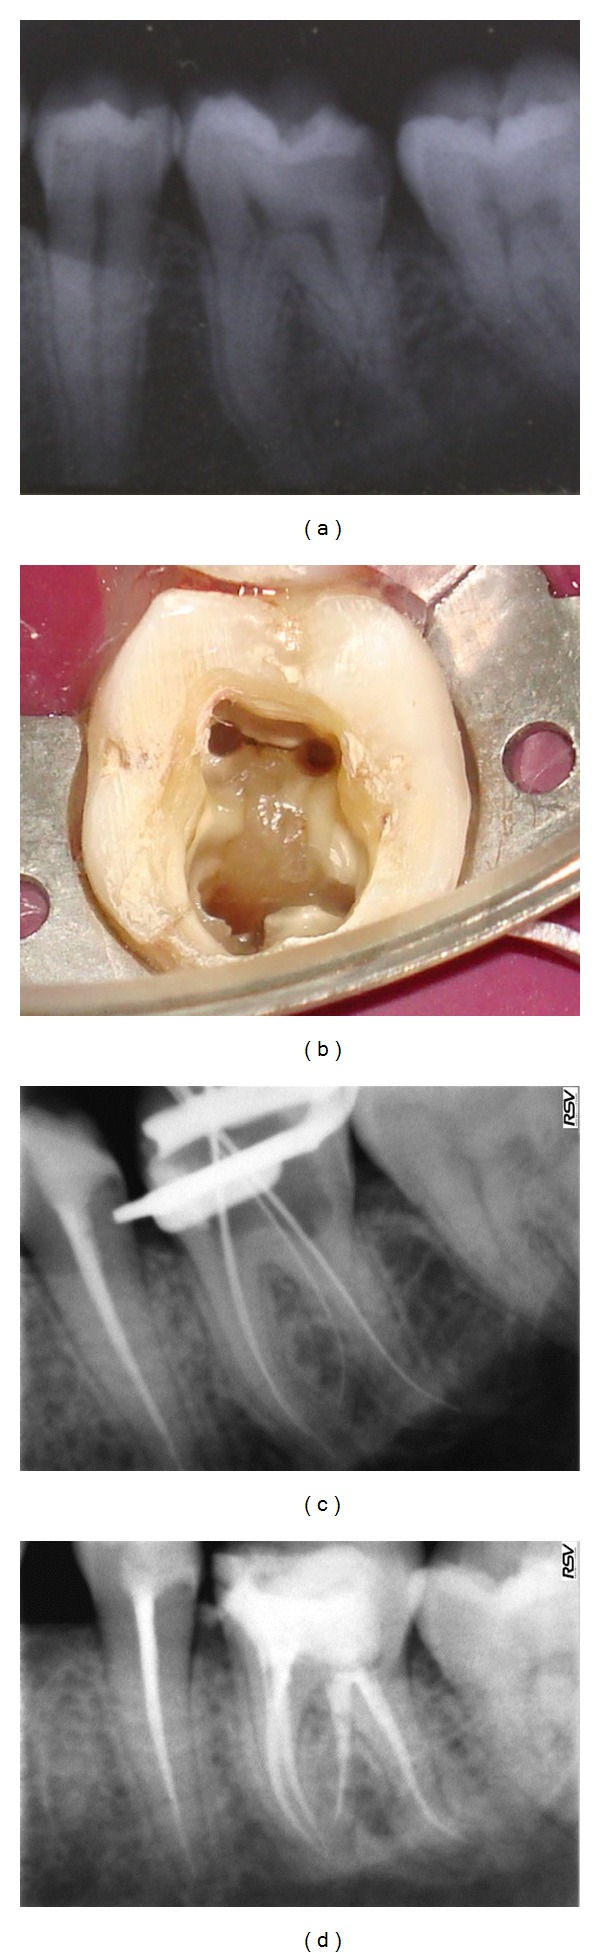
(a) Diagnostic radiograph, (b) access cavity preparation, (c) working length determination, and (d) postfilling.
